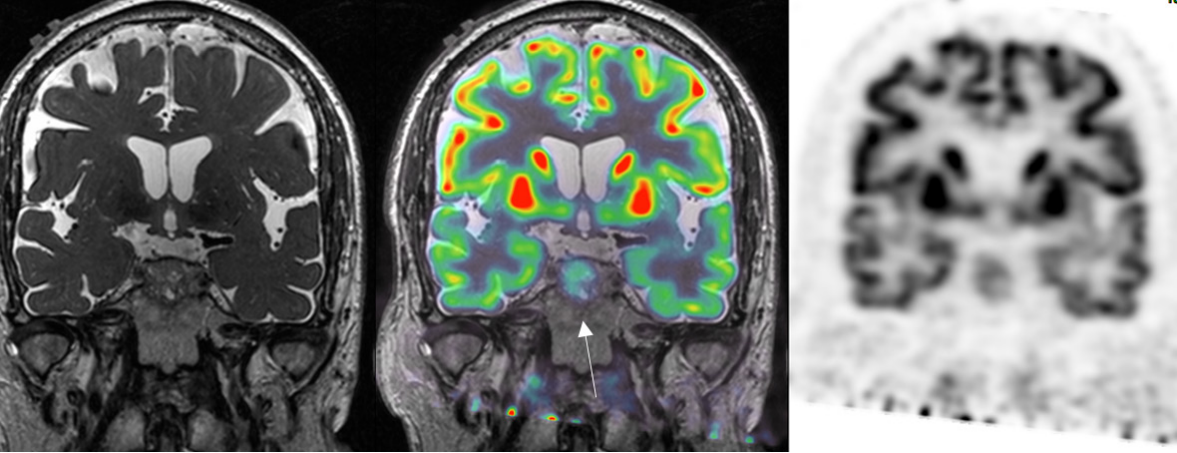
Coronal PET‑CT F18‑FDG with MRI coregistration: moderate uptake of infiltrative sellar lesion. Moderate FDG uptake of infiltrative sellar lesion.
